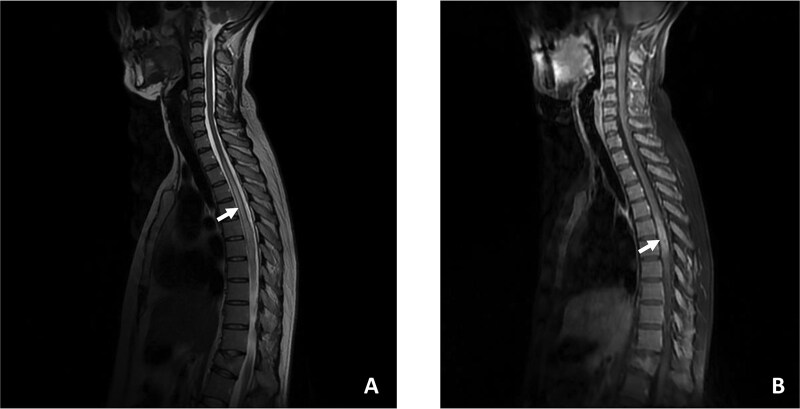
T2-weighted magnetic resonance image showing an extensive hyperintensity from D1 to D8 (A), with gadolinium enhancement (B).
